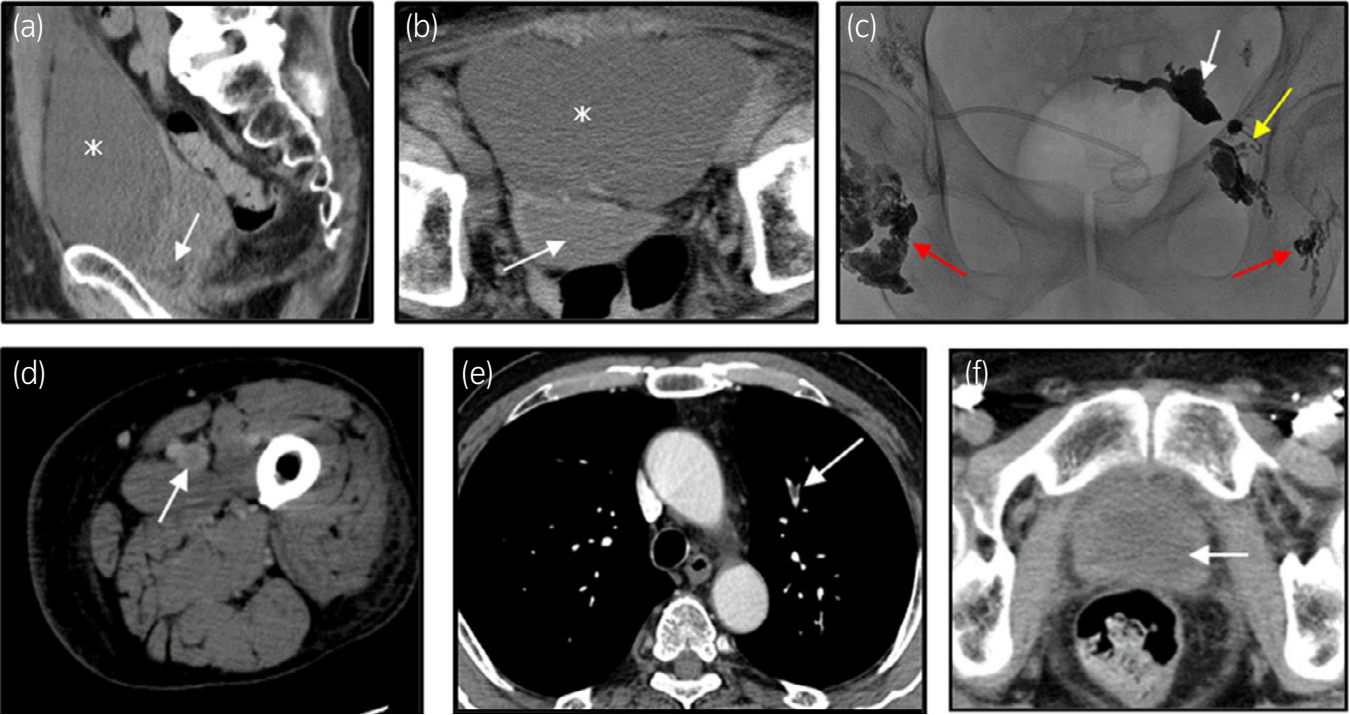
Representative images of Case 1 during treatment. Abdominal CT shows a large lymphocele in front of the bladder (a: sagittal image, b: axial image. The asterisk indicates the lymphocele; the white arrow indicates the bladder). The left lower extremity CT shows a deep vein thrombosis (c: axial image, white arrow). The pulmonary artery CT shows pulmonary vein thrombosis (d: axial image, white arrow). The representative lymphangiography image of Case 1: The left side of the lymphangiography shows lymphatic leakage in the upper stream of the lymphatic vessel (e: red arrows indicate the bilateral inguinal nodes; yellow arrow indicates the left lymphatic vessel; and white arrow indicates the lymphatic leakage site). Abdominal CT was performed 50 days after RRP. The lymphocele had completely disappeared (f: axial image, white arrow indicates the bladder).
